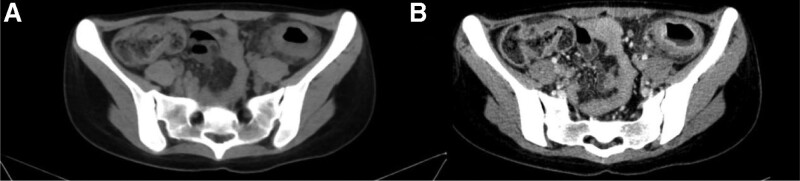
The descending and sigmoid colon narrowed (A) as seen in the abdominal CT. Colorectal wall thickening, mural enhancement and stratification is observed on the enhanced scan (B). CT = computed tomography.
